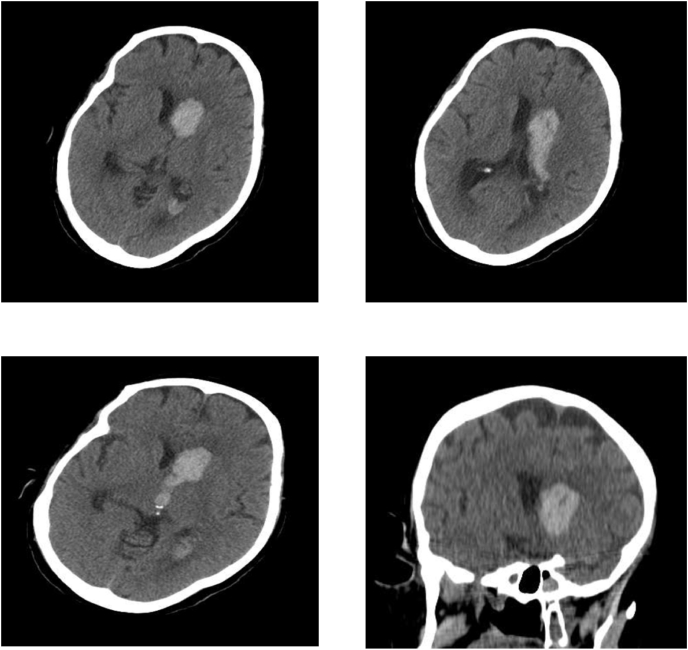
Axial non contrast brain computed tomography demonstrating a large parietal hyperdense hemorrhage measuring 40 × 15mm surrounded by a small area of peri-lesional edema with compression of the homolateral lateral ventricle associated with an intra ventricular hemorrhage.
